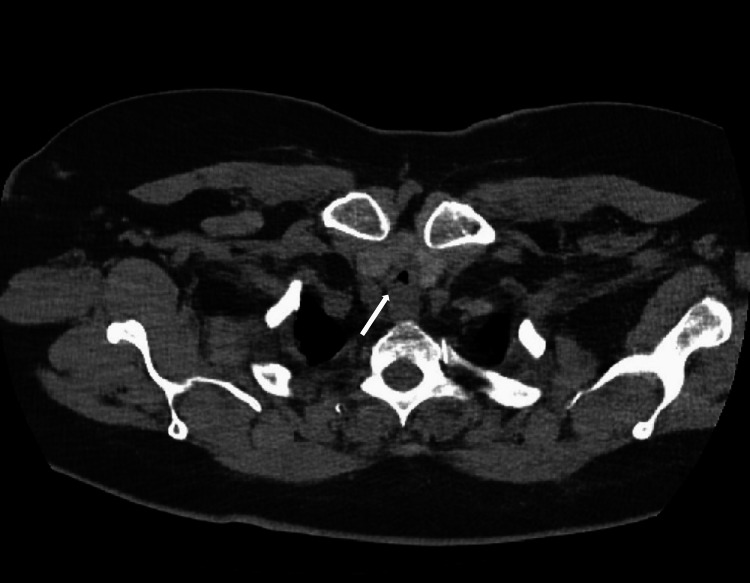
Axial view of computed tomography scan of chest without contrast showing subglottic concentric tracheal stenosis measuring 5.57 cm x 5.20 cm in dimension
